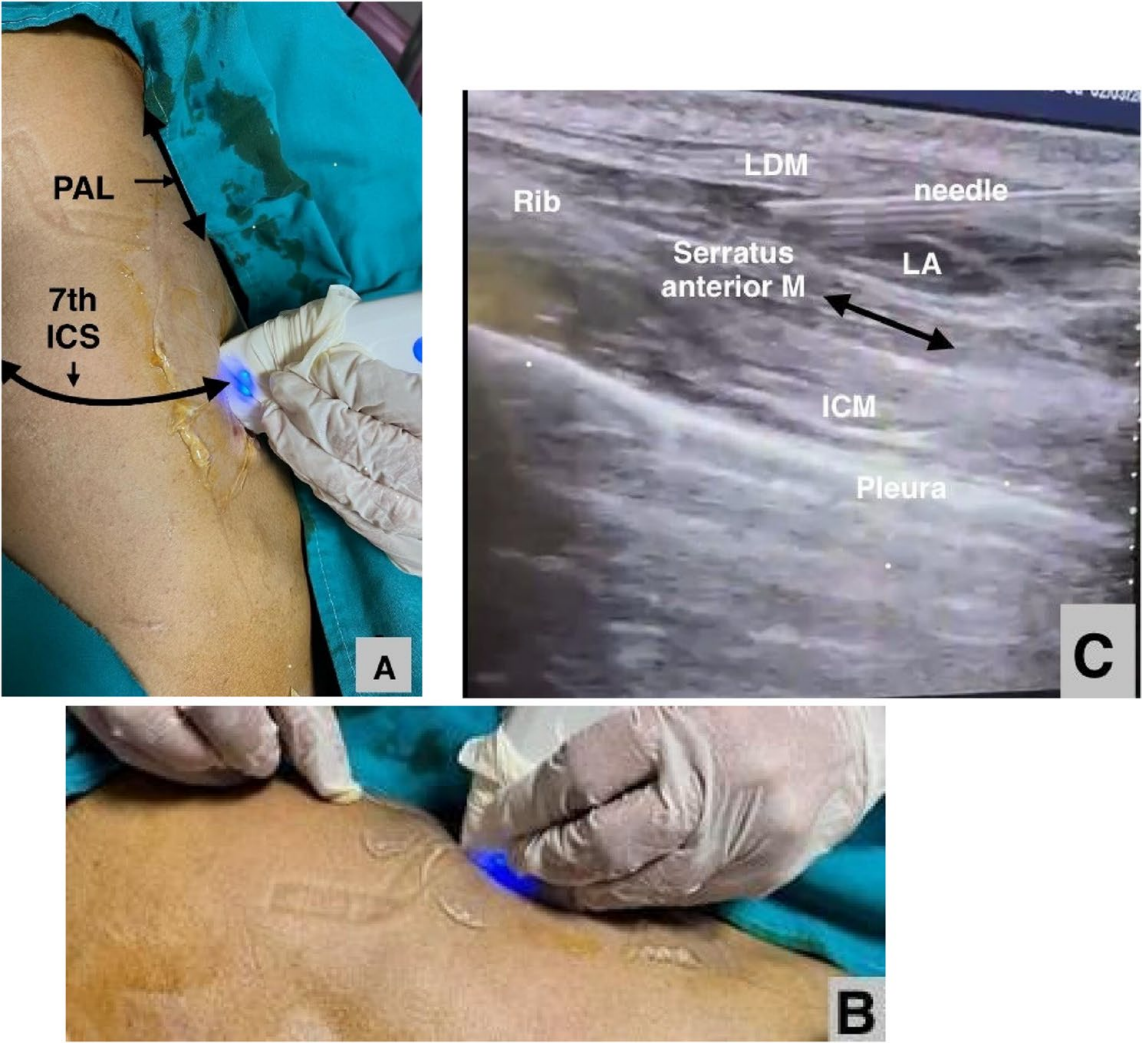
(A) Patients in lateral decubitus, the straight arrow represents posterior axillary line (PAL) and the linear probe held in longitudinal plane overlying the 7^th^ intercostal space (7^th^ ICS) (B) The block needle inserted in craniocaudal direction (C)Sonographic image of the modified serratus anterior plane block. LD: latissimus dorsi muscle, ICM: intercostal muscle, LA: local anesthetic spread between the serratus, and LD muscles
